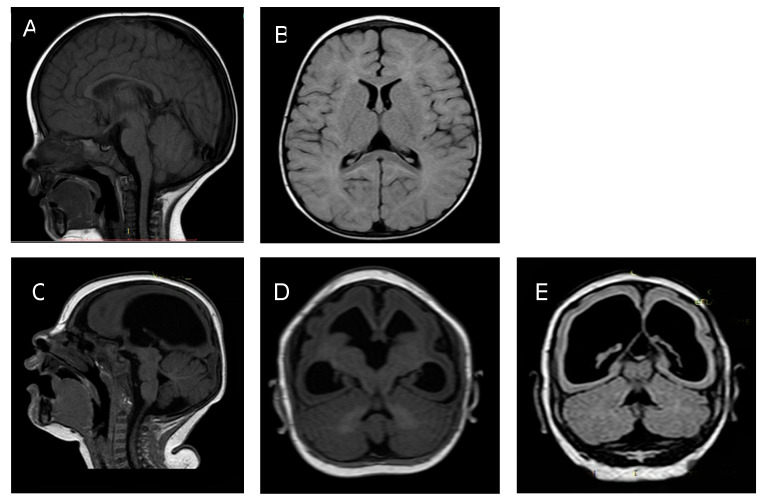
Twin A: Panel A: Brain MRI at 7 months of age sagittal T1 showing normal brain cortex formation and thickness, normal corpus callosum, and posterior fossa. Panel B: Axial T2 Fluid Attenuated Inversion Recovery (FLAIR) showing normal ventricular size. Twin B: Panel C: Brain MRI at 7 months sagittal T1. Panel D axial T2 FLAIR. Panel E axial T1. Images show a thin cortical mantle, ex vacuo enlargement of lateral ventricles, reduced cortical folds, and hypogenesis of the corpus callosum.
